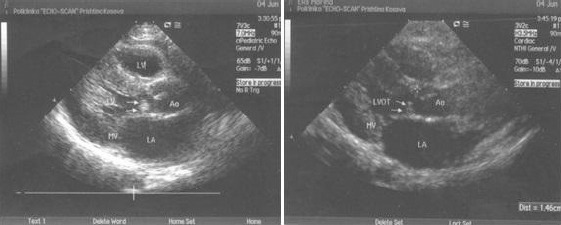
Transthoracic view of eight years old boy, previously operated from perimembranous VSD, showed subaortic membrane few millimeters under arotic valve, closing left ventricular outflow tract*.
